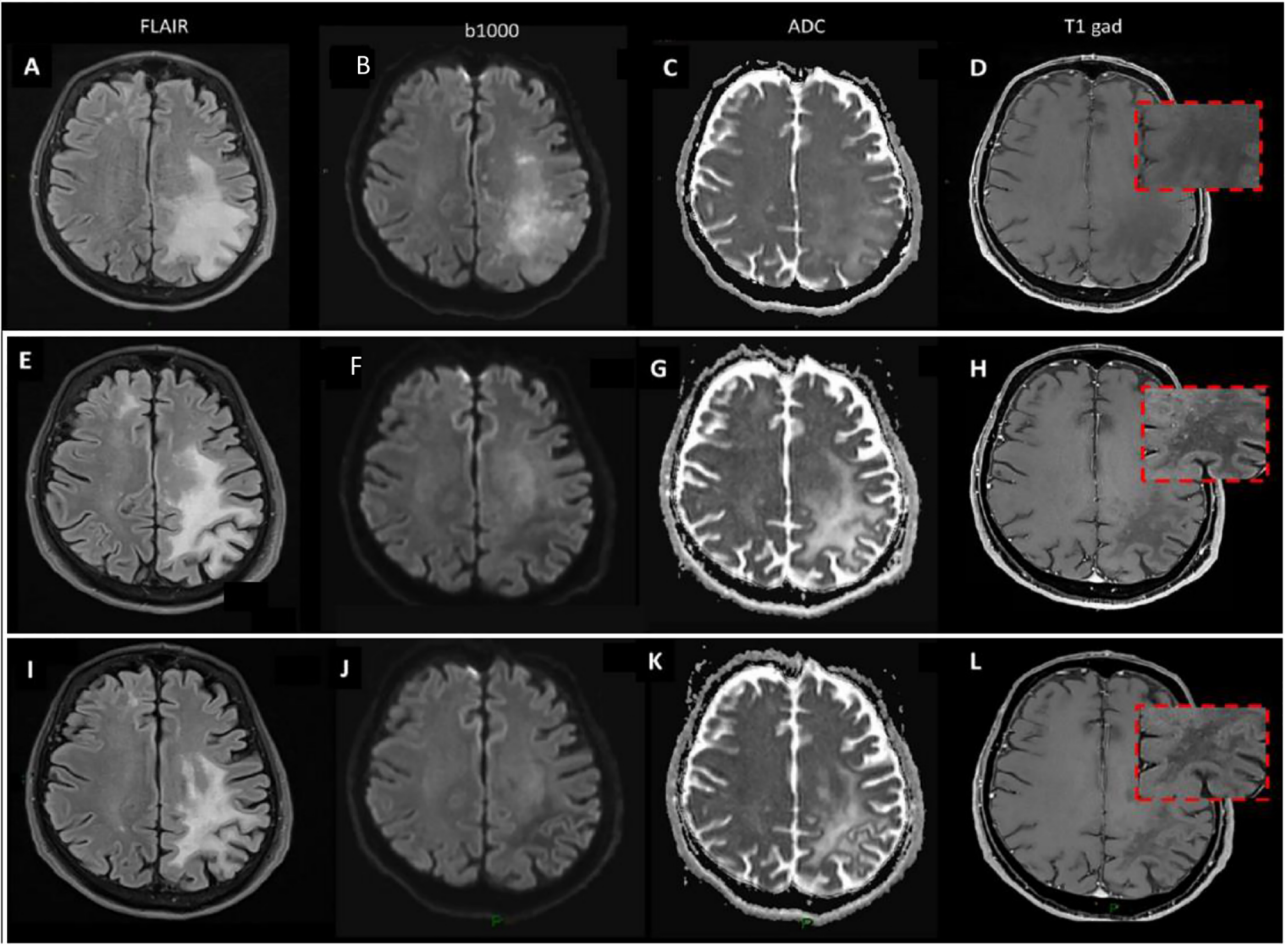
Brain MRI. (A–D) December 2017 brain MRI at PML diagnosis. (E–H) May 2018 brain MRI during ICI treatment. (I–L) January 2019 brain MRI after ICI treatment.
